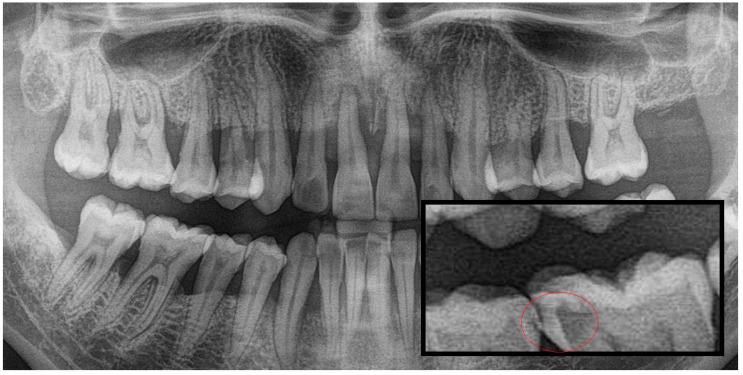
Panoramic radiography and segmented interproximal caries, red circle shows the caries.
